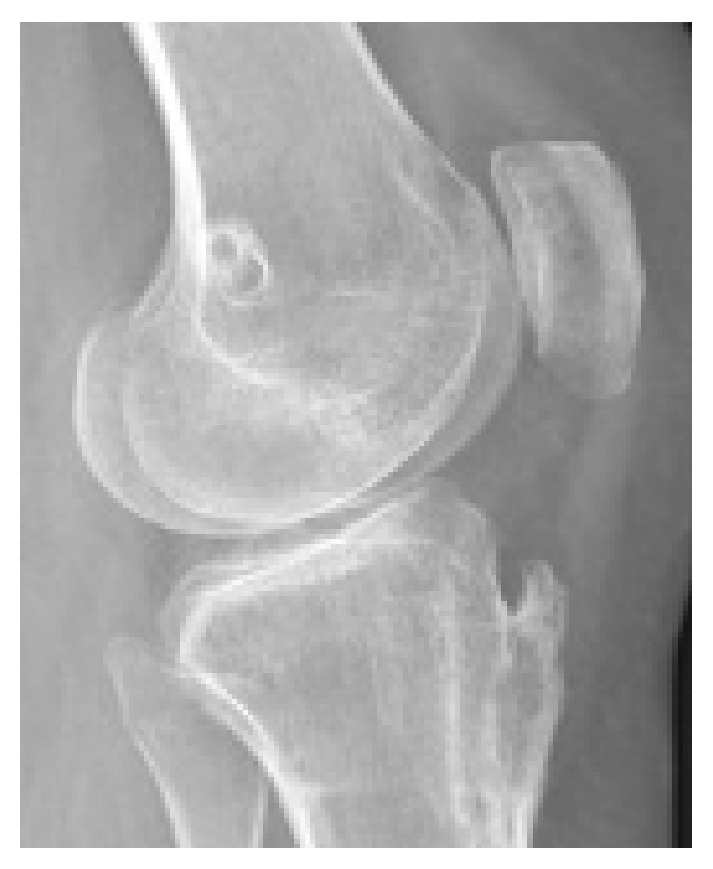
Post-op lateral knee X-ray.
